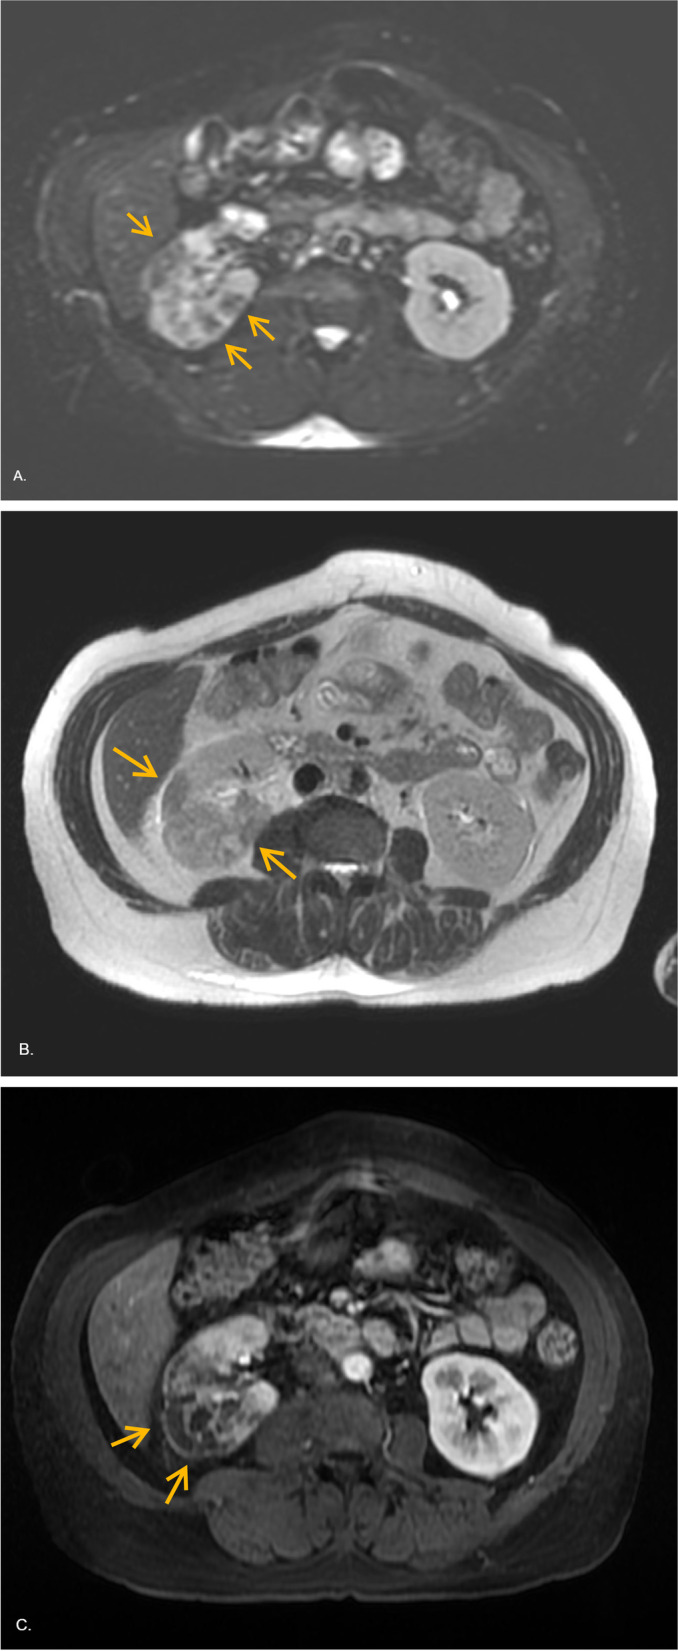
A DWI demonstrated no diffusion restriction (arrows). B T2 weighted image showed hypointense signal compared with normal parenchyma (arrows). C Post contrast T1 weighted image showed wedged shaped infarcted areas with low signal intensity with obliteration of corticomedullary differentiation of right kidney (arrows)
